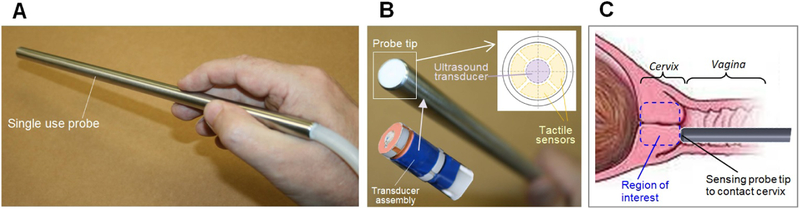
Cervical probe. (A) General view of the Cervix Monitor probe; (B) Probe tip with tactile and ultrasound transducers; (C) Probe positioning at cervical measurement.
